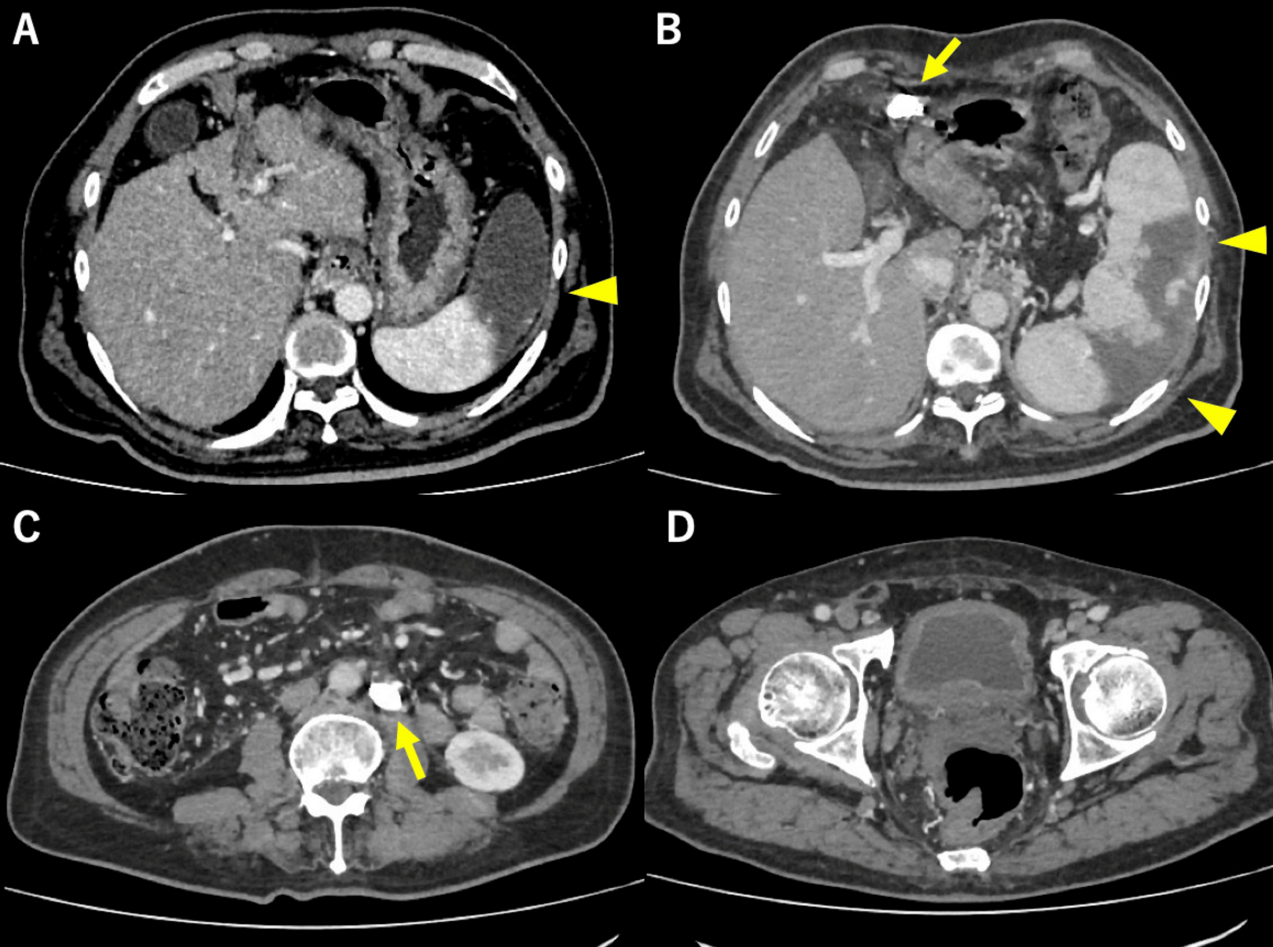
CECT after the second BRTO. Portosystemic shunts were embolized with coils (yellow arrows), and partial splenic embolization was performed (yellow arrowheads). (A) Partial splenic embolization is seen (arrowhead). (B) Coil in the paraumbilical vein (arrow) and splenic embolization (arrowheads). (C) Coil in the mesorenal shunt (arrow). (D) No residual shunts in the lower abdomen. CECT: Contrast-enhanced computed tomography; BRTO: balloon-occluded retrograde transvenous obliteration
